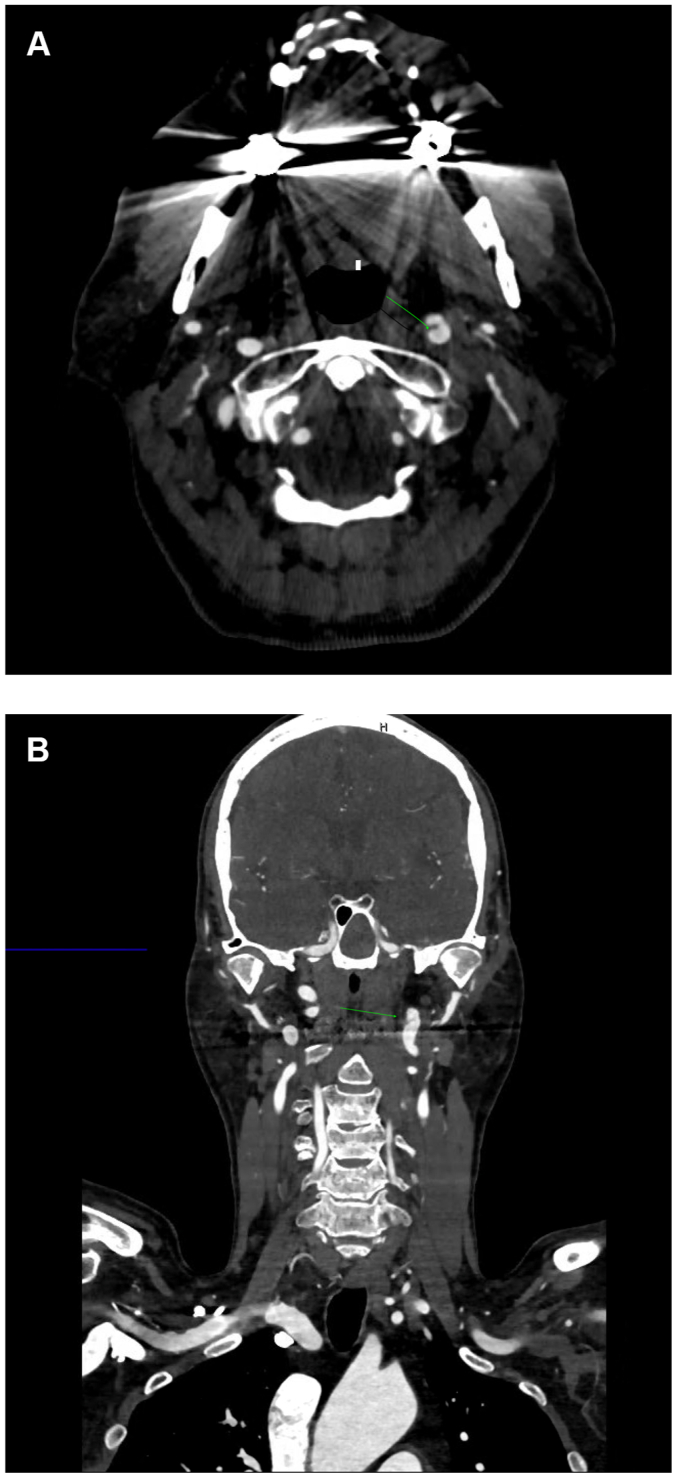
Computed tomography angiogram (CTA) transverse (A) and coronal (B) views showing left internal artery dissection, *green arrows*.
